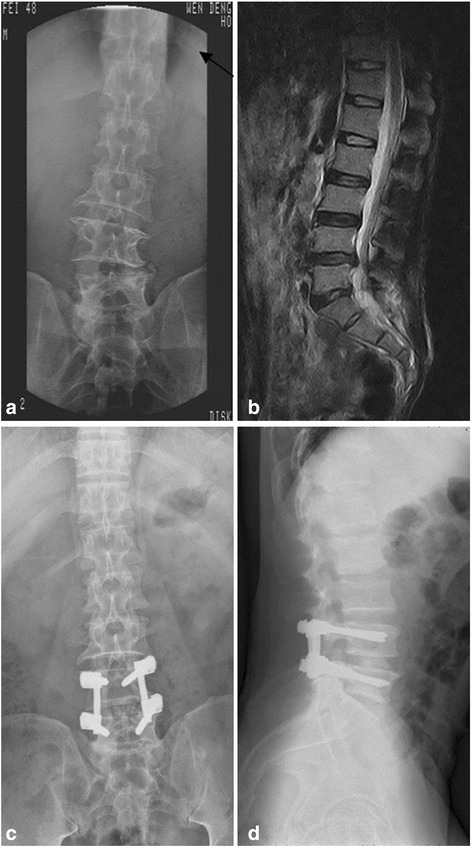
A 72 year old male patient with back pain and intermittent claudication for a year. a) preoperative radiograph showing degenerative scoliosis with 15° Cobb angle, L3/4 interval tip angle, 0.34 of W/AL. b) preoperative MRI showing L4/5 disc herniation, spinal stenosis, and degenerative L4/5 instability. c) postoperative anteroposterior radiography showing good fixation and fusion without secondary scoliosis. d) postoperative lateral radiography showing L4/5 segment fused and satisfying lumbar lordosis
